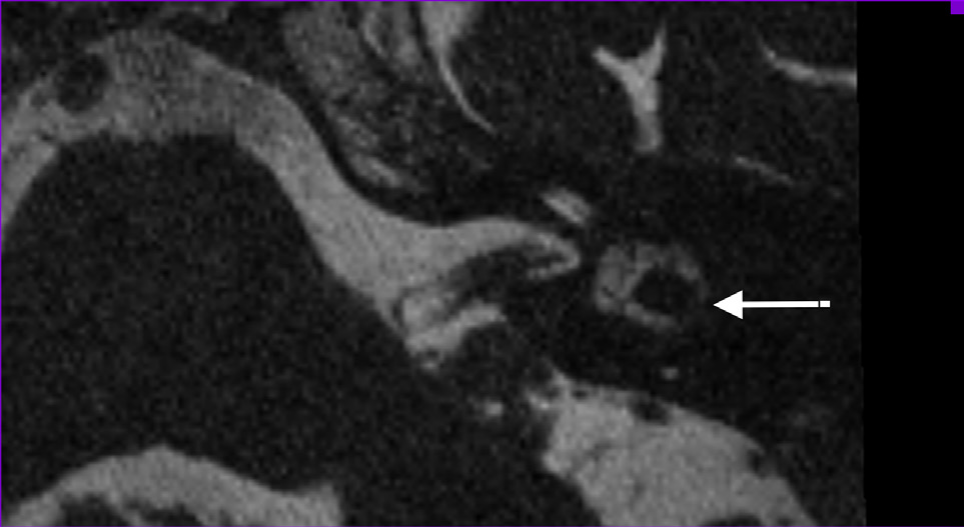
Axial T2-weighted turbo spin echo MRI of the left labyrinth demonstrating the signal loss in the lateral semicircular canal (arrow) corresponding to the axial CT-slice in Figure 1.
